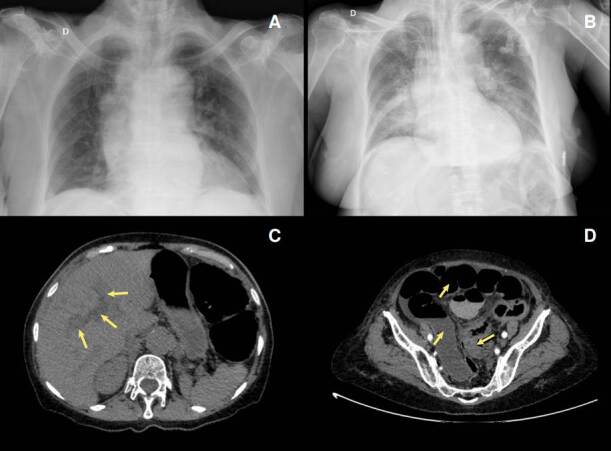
A) Chest X-ray shows cardiomegaly and bilateral hilar lymph node enlargement. B) Chest X-ray, taken 24 hours after the initial test, indicates cardiomegaly and bilateral alveolar infiltrates predominantly on the right side. C) Simple and contrasted abdominal computed tomography showing intrahepatic dilated bile duct (arrow). D) Marked distension of the intestinal loops (arrow), with thickening, showing a decrease in the intestinal lumen at the sigmoid colon (arrow).
